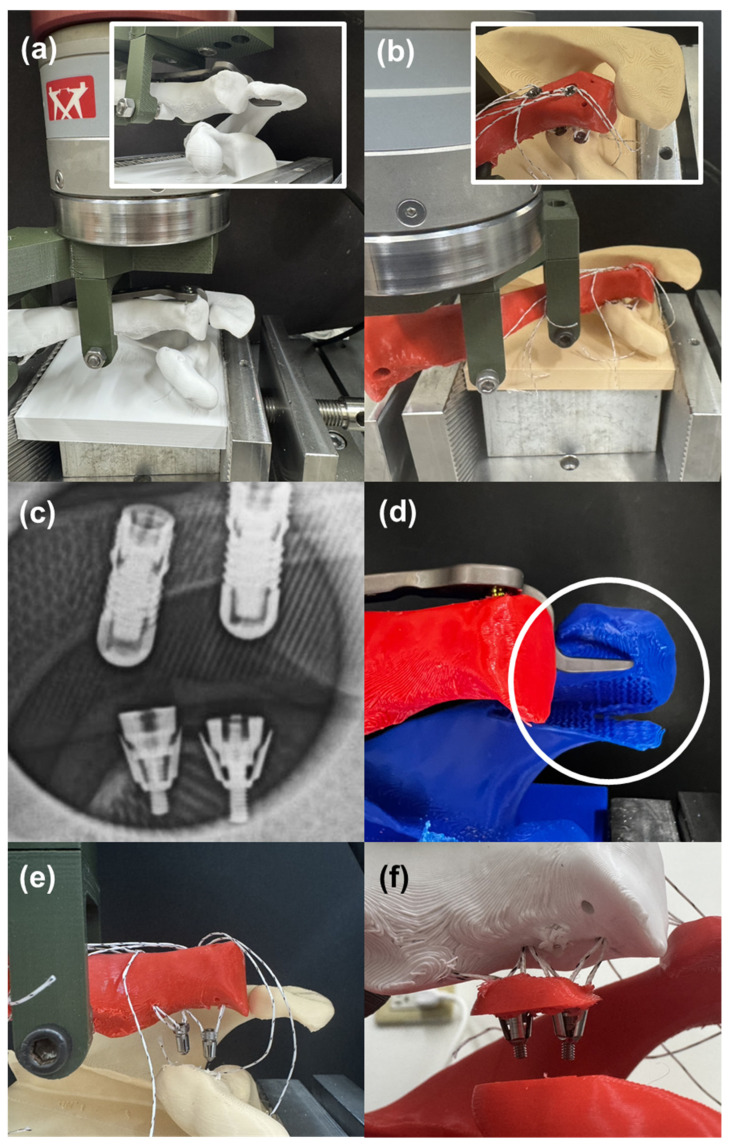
Coracoclavicular (CC) ligament reconstruction model and fixation outcomes. (a) Experimental setup using a traditional hook plate clasped beneath the acromion. (b) Fixation with the SEW anchor implanted into the coracoid process. (c) Radiograph confirming successful deployment of SEW anchor wings after implantation. (d) Hook plate fixation resulting in acromial fracture and structural disintegration. (e) SEW-NP anchors (wings not deployed) showing individual anchor pull-out failure. (f) SEW-OP anchors (wings deployed) resisting individual pull-out and instead failing together with the coracoid bone block, demonstrating superior fixation strength.
